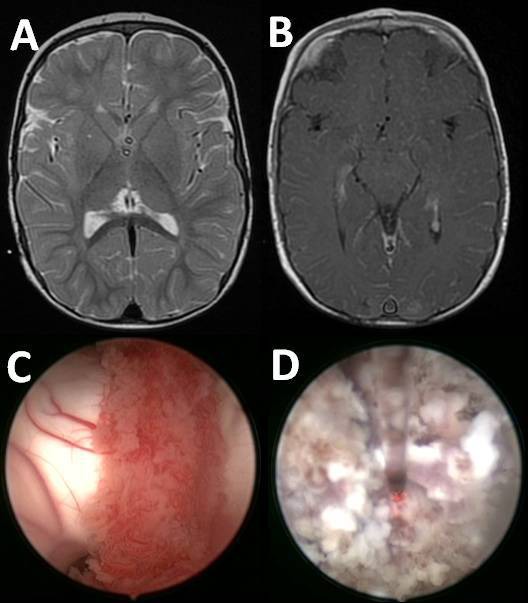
Brain magnetic resonance imaging (MRI) after Gadolinium administration ruled out pathological conditions affecting the choroid plexuses (A),(B). Endoscopic pictures of the choroid plexus before (C) and during (D) the coagulation performed using the Thulium laser.
